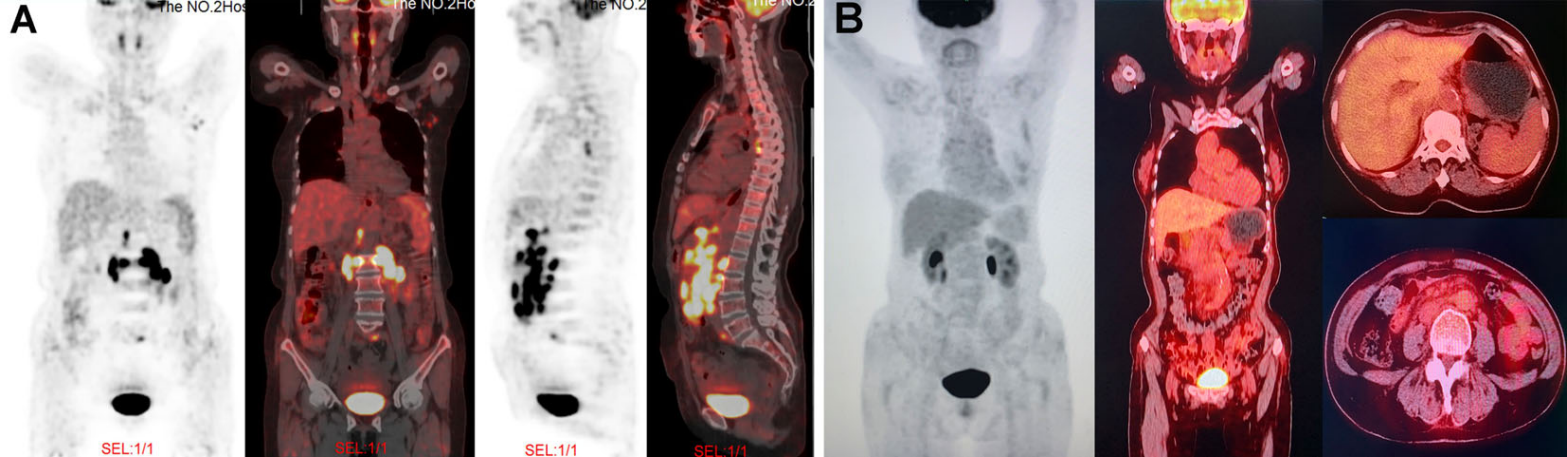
PET/CT images at different time points during treatment. (A) At the time of diagnosis, PET/CT revealed the lymphadenopathy in the mediastinum, left axilla, peritoneal retroperitoneal, bilateral diaphragmatic foot, and left peri-iliac vessels; as well as several nodular FDG-avid lesions in the right scapula with hypermetabolic activity (SUVmax: 7.9). (B) After four cycles of GemOx plus tislelizumab combination treatment, PET/CT revealed that patient achieved complete response.
